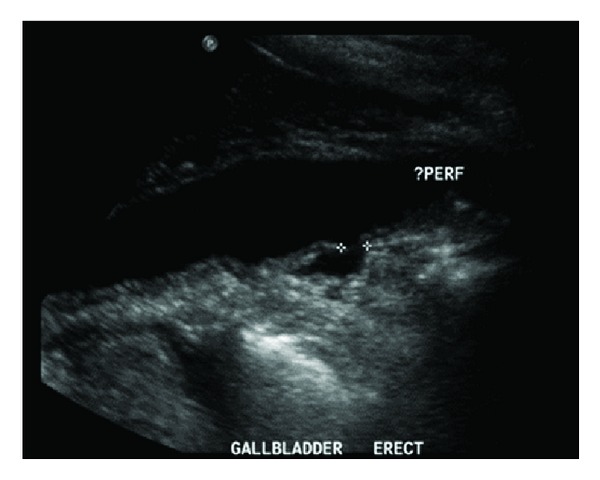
Ultrasonographic evidence from the repeat ultrasound of gallbladder perforation.
